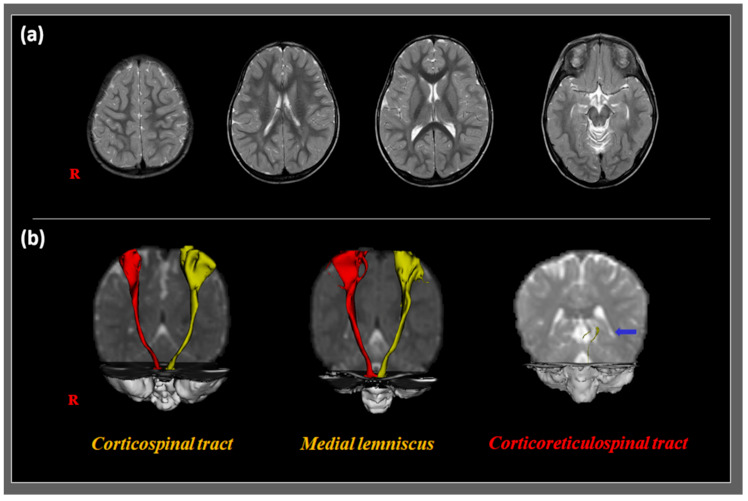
T2-weighted magnetic resonance images (upper row) and diffusion tensor tractography (DTT) images (lower row) of a 23-month-old boy with developmental coordination disorder showing gait dysfunction. (a) T2-weighted magnetic resonance images show no abnormal lesions. (b) DTT images revealing a disrupted corticoreticulospinal tract (blue arrow). However, the corticospinal tract and medial lemniscus show preserved integrity.
